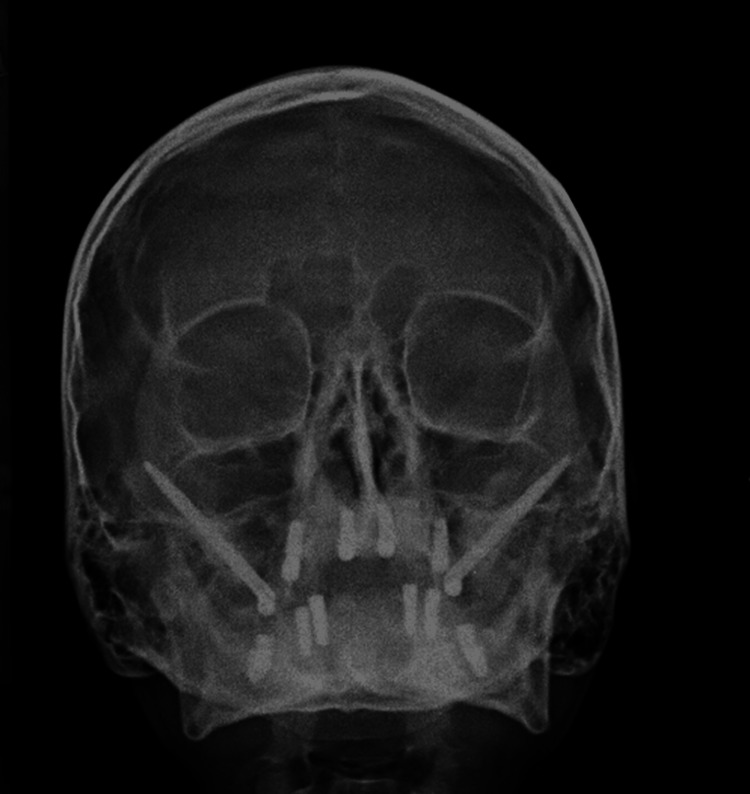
Postoperative PNS X-ray of the patient Image credit: Dr. Bhushan Mundada PNS: Paranasal sinus
